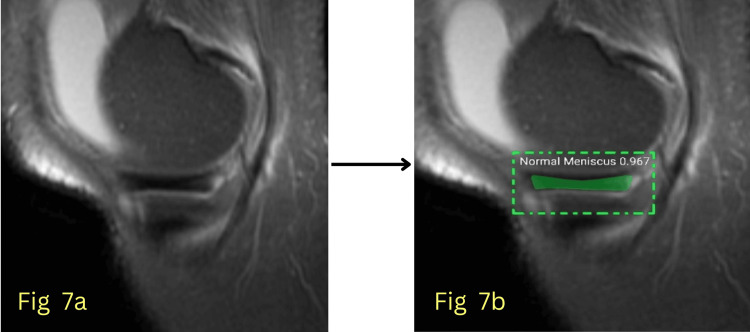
(a) Input MRI image; (b) Segmented image showing the normal body of the meniscus.
